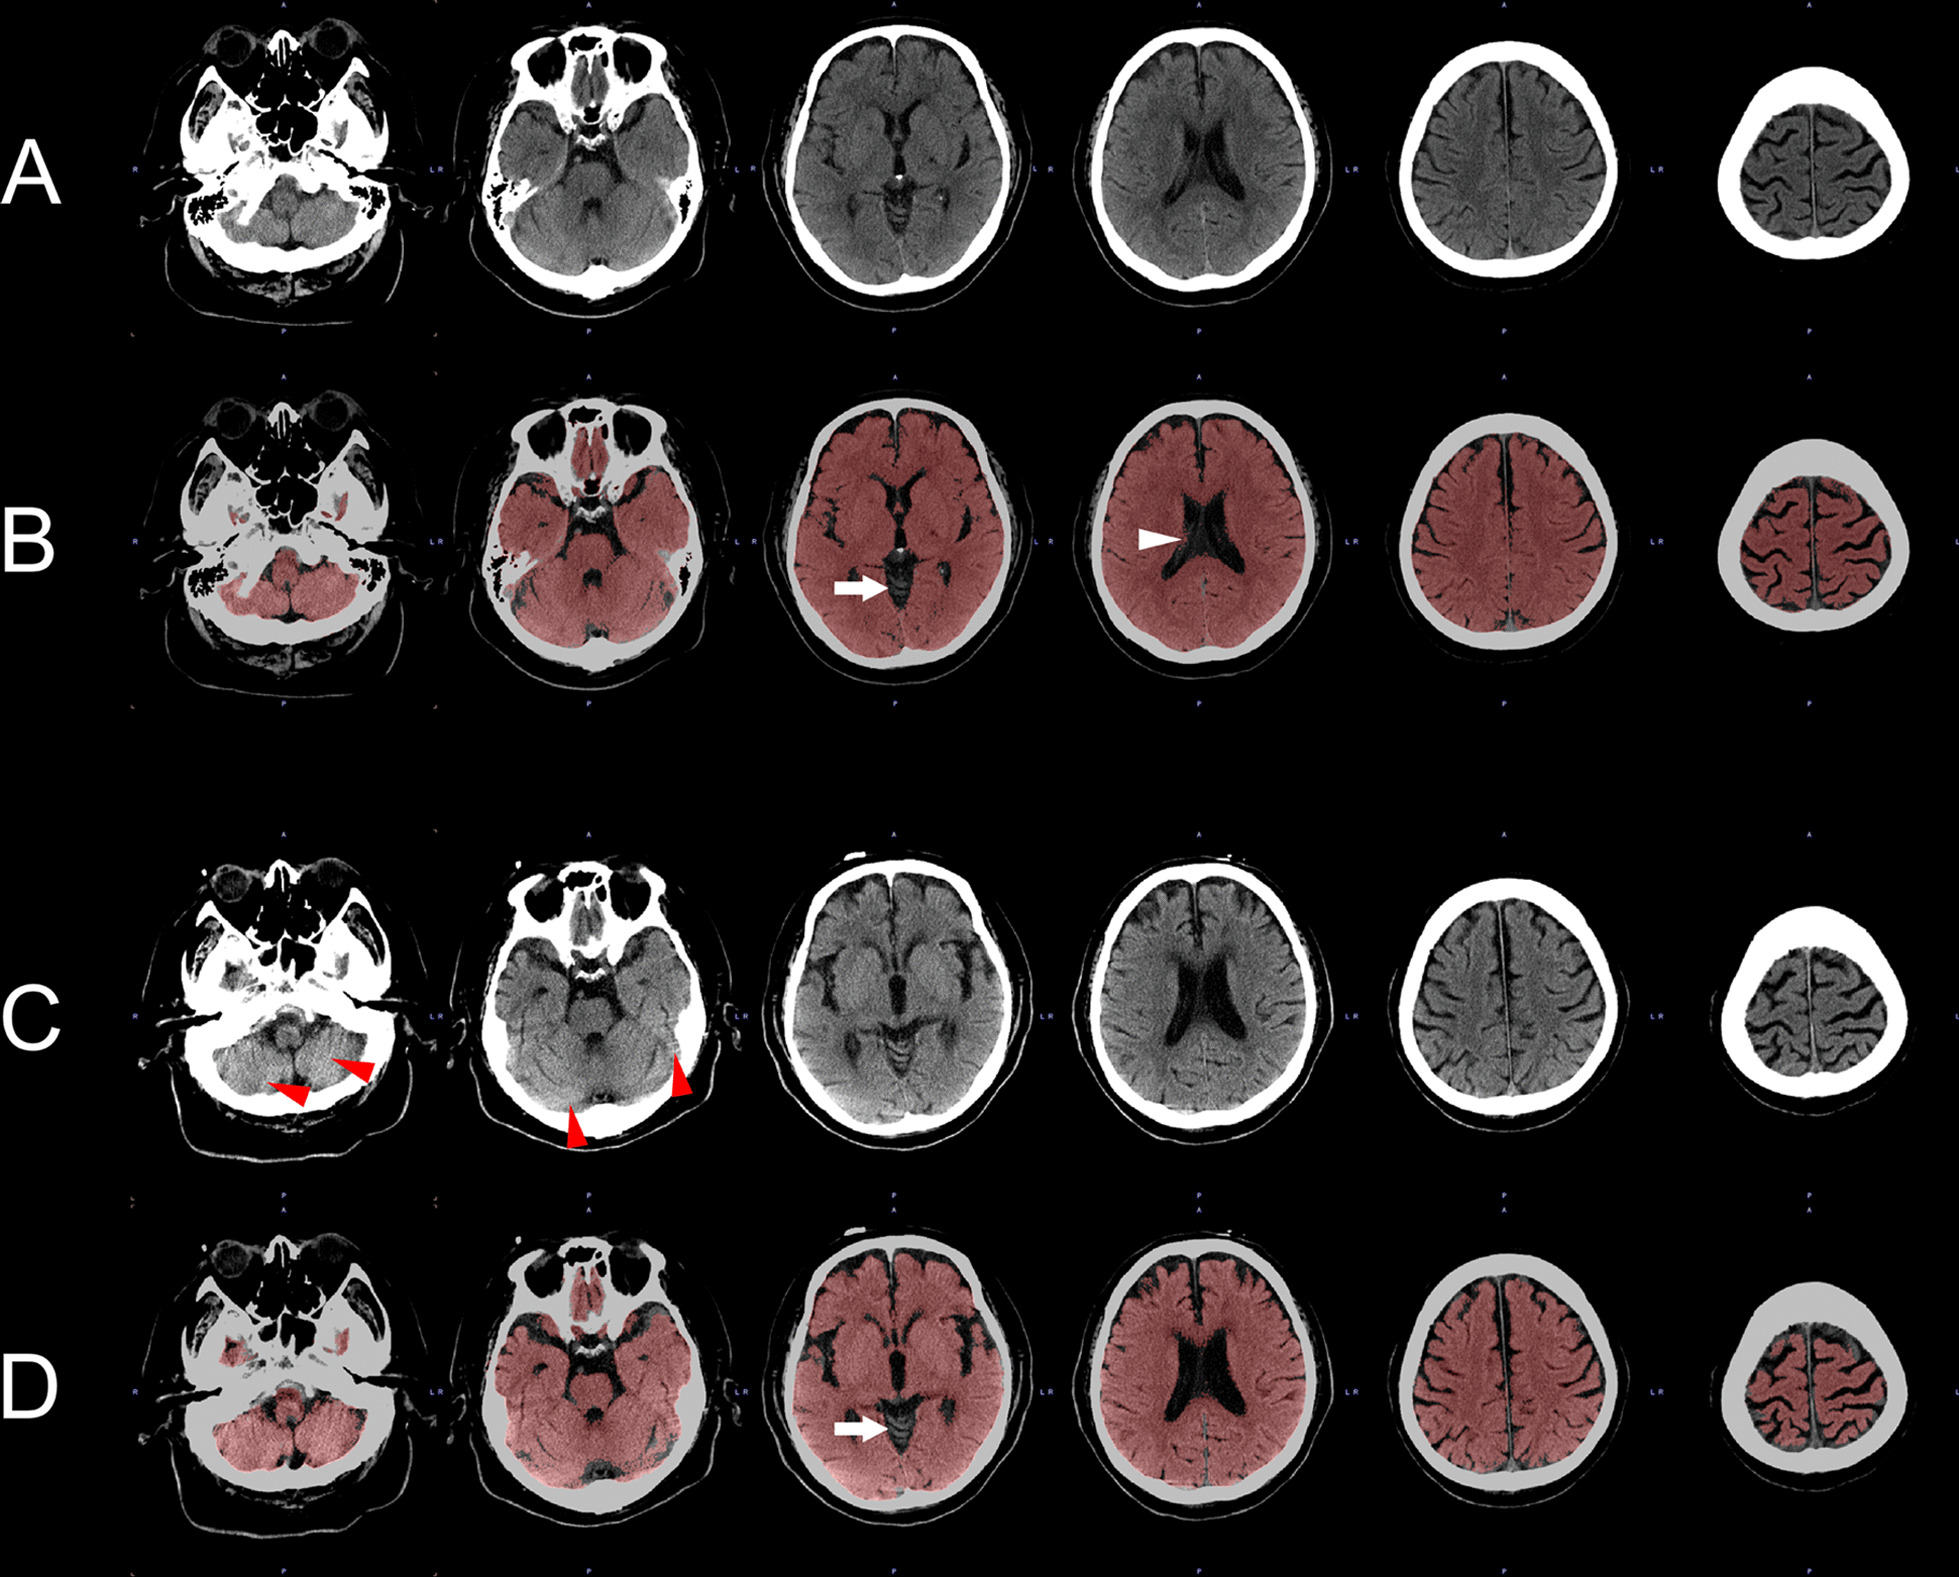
Axial view of brain computed tomography (CT) scan of a 63-year-old man who was hospitalized and treated for 107 days for sepsis due to soft tissue infection. A and B are the first CT scan and C and D are the second CT scan of the patient. A and C are original brain CT scans and B and D are brain CT scans with a corresponding segmentation map where gray and white matter are colored red. Compared to the first CT scan (A), atrophy is evident on the second CT scan (C). Although small misclassifications are observed in the cerebellar vermis (arrows on B and D) and the right choroid plexus (white arrowhead on B), automatic segmentation was successful. Subtle beam-hardening artifact is found around the posterior fossa (red arrowheads on C),; it did not affect the classification. A 7.4% decrease in brain volume was calculated by the automatic algorithm
